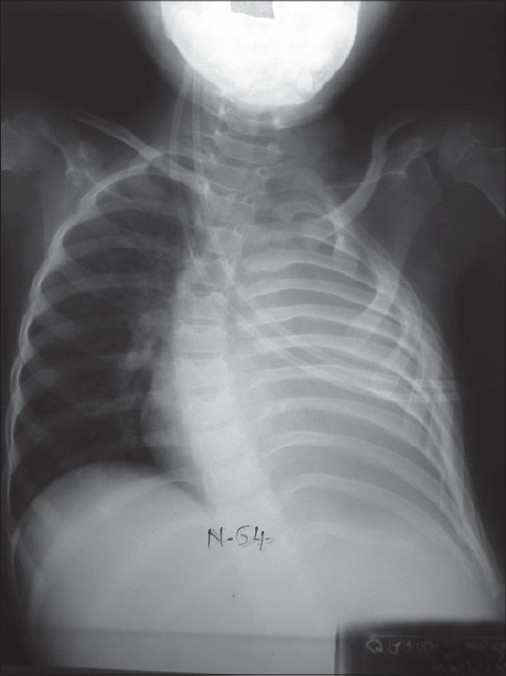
Chest X-ray PA view showing complete opacity on left side and scoliosis
